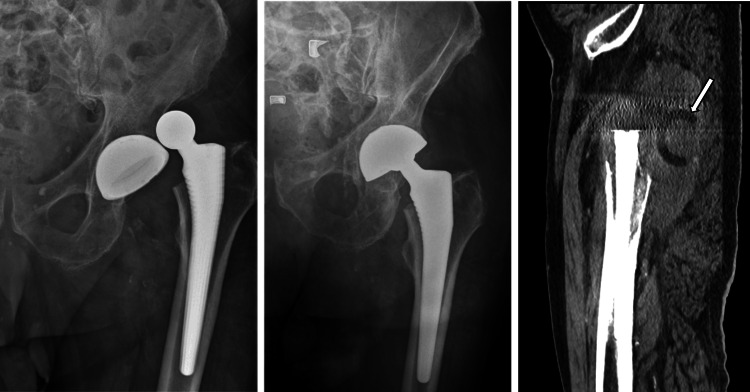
Post-reduction hip radiograph demonstrating eccentric femoral head position. Sagittal CT image confirms polyethylene liner dissociation with migration into the peritrochanteric soft tissues - “bubble sign” (arrow). CT, computed tomography.
